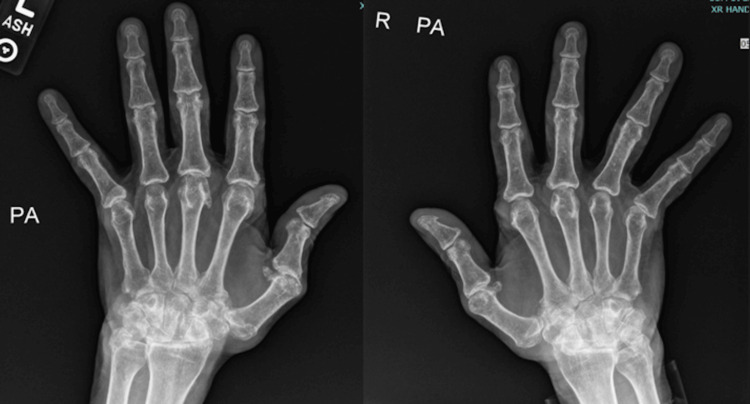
X-ray hands revealing subluxation of the metacarpophalangeal joints.
